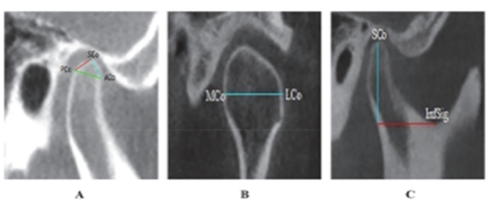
Cone beam computed tomography images images showing condylar morphology measurements: (A) condylar length between ACo–PCo, (B) condylar width between MCo–LCo, and (C) condylar height from SCo to the sigmoid notch. ACo: anterior condyle point; PCo: posterior condyle point; MCo: medial condyle poles; LCo: lateral condyle poles: SCo: superior condyle point.
